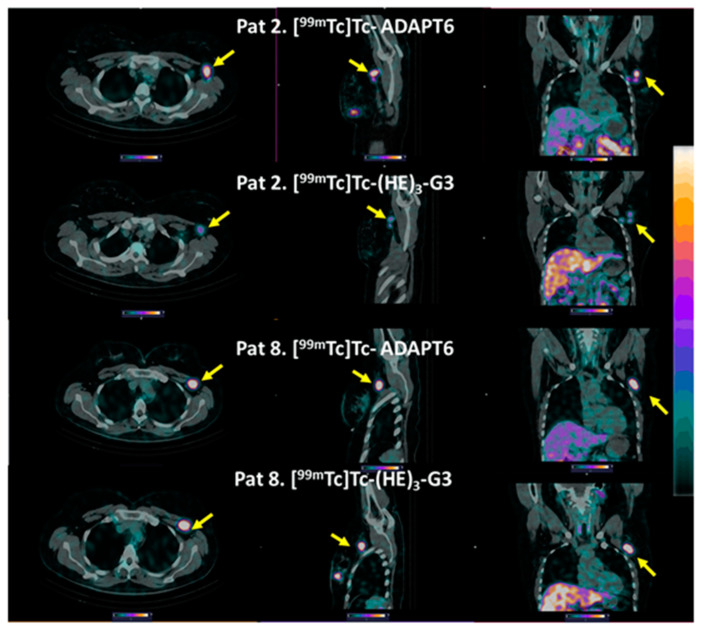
Representative SPECT/CT images of HER2-positive lymph node metastases after injection of [^99m^Tc]Tc-ADAPT6 (2 h after injection) and [^99m^Tc]Tc-(HE)_3_-G3 (4 h after injection). Arrows point at metastases. The upper setting of a linear intensity scale is adjusted to SUV 6.8 in all images.
